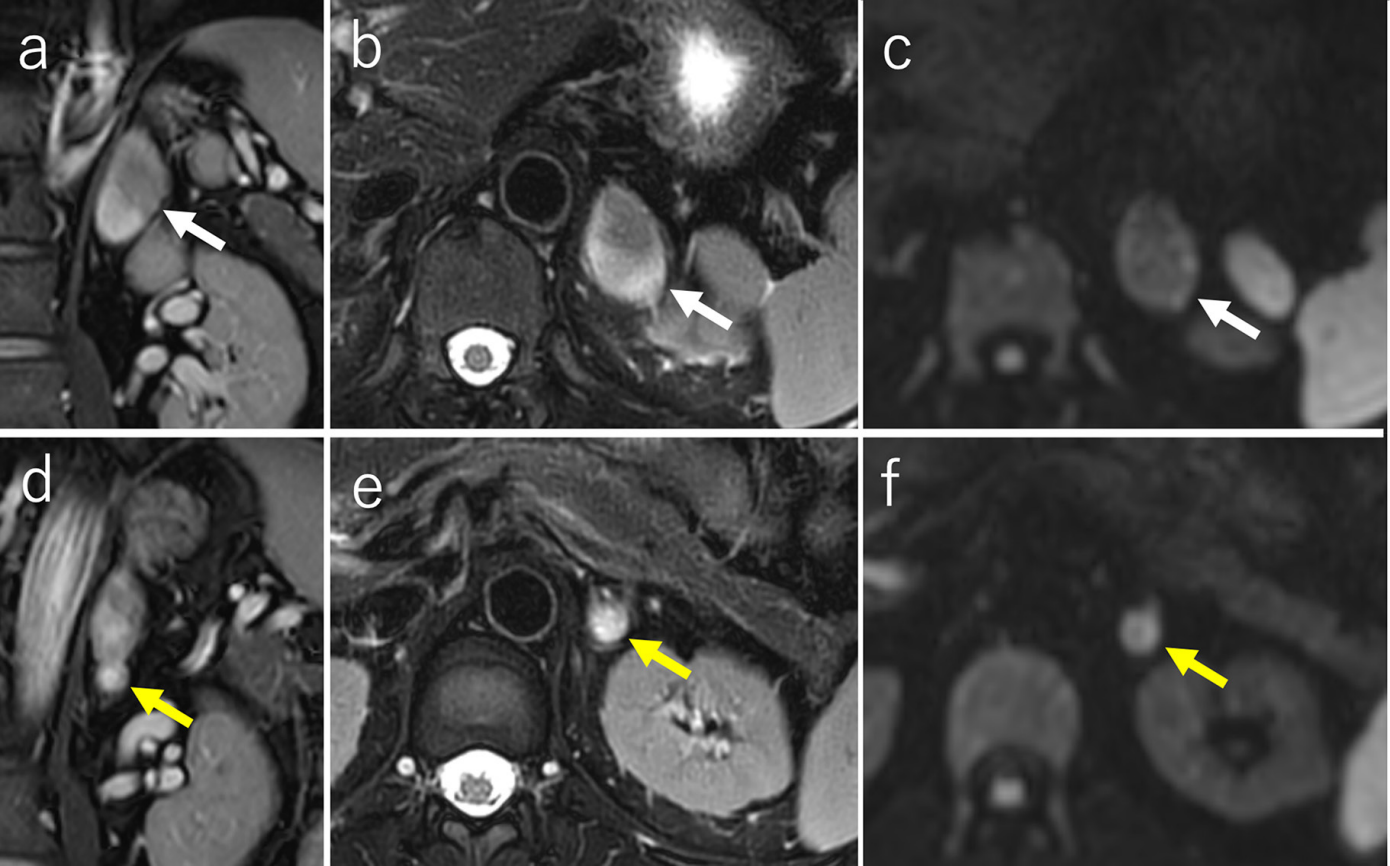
MRI. (a–c) MRI of the cephalic mass in the left adrenal gland (white arrows). Coronal (a) and axial (b) fat-suppressed, T2 weighted images show the cephalic lesion with a mixture of high and low signal intensities and curvilinear bands of low intensity. (c) Diffusion-weighted image shows slightly high signal intensity. (d–f) MRI of the caudal nodule of the left adrenal gland (yellow arrows). Coronal (d) and axial (e) fat-suppressed, T2 weighted images show the caudal nodule with heterogeneous, high signal intensity. (f) Diffusion-weighted image shows high signal intensity.
